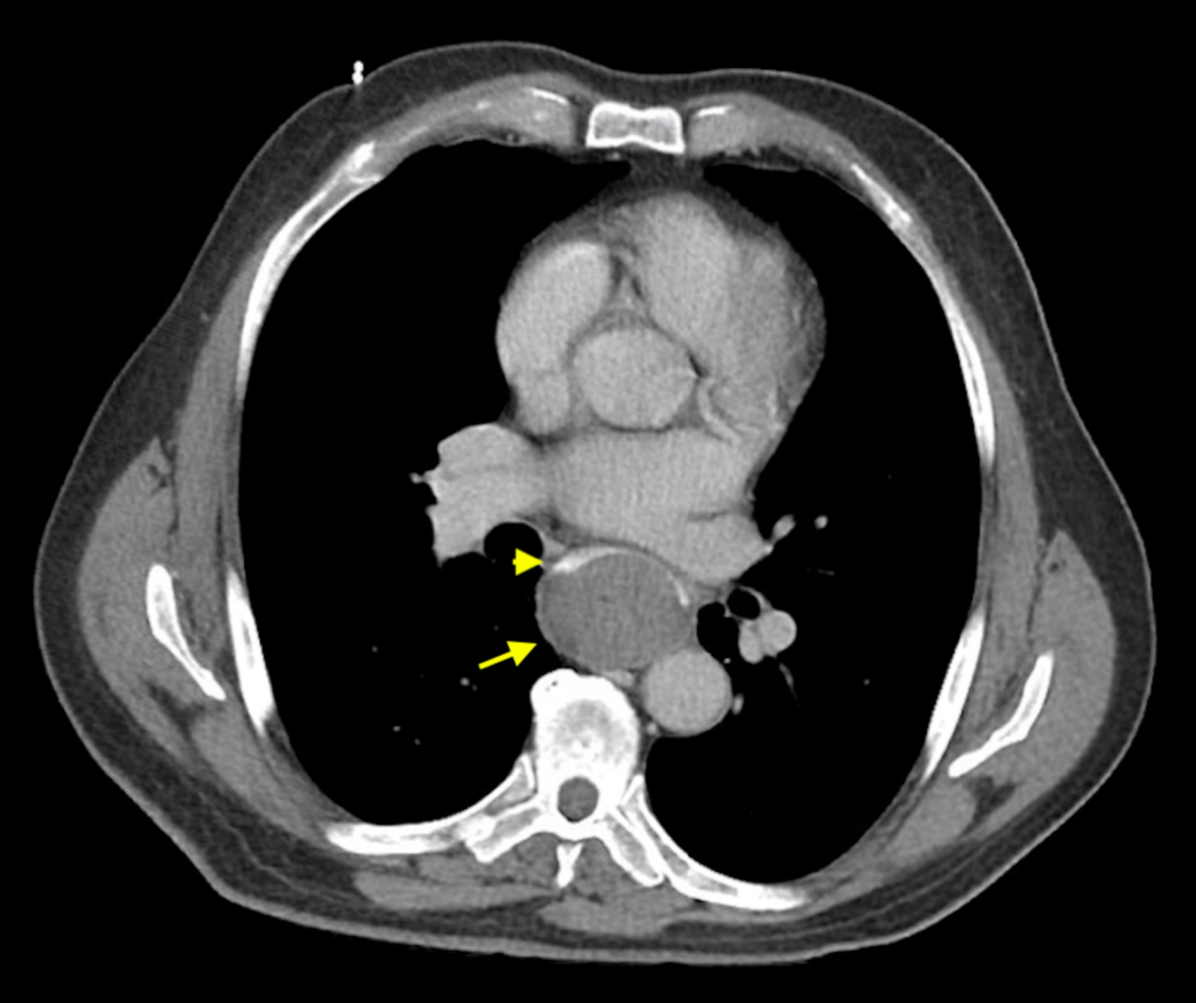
Computed tomography (CT) abdomen with oral and intravenous (IV) contrast showing hyperdense oral contrast layering (yellow arrowhead) over a rounded, well-circumscribed structure in the visualized distal esophagus (yellow arrow) concerning for an intramural esophageal hematoma
